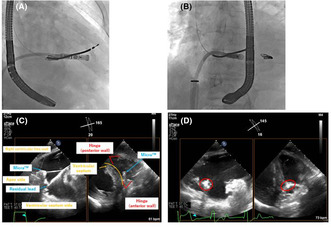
With regard to the leadless pacemaker (Micra™) implantation (LPI) procedure, it is important to avoid cardiac tamponade. It is desirable to confirm that the Micra™ (Medtronic) tip faces the ventricular septum by using sufficient contrast medium in addition to the fluoroscopic image of right anterior oblique (RAO) and left anterior oblique (LAO) view. However, in patients with poor renal function or contrast agent allergy, placement using a sufficient amount of contrast agent is difficult, and placement using an intracardiac echo guide has been reported.^1^ In this case, a patient with mitral regurgitation (MR) and sick sinus syndrome (SSS) with severe renal impairment underwent transcatheter mitral valve repair (TMVr) and LPI under transesophageal echocardiography (TEE) guidance.
